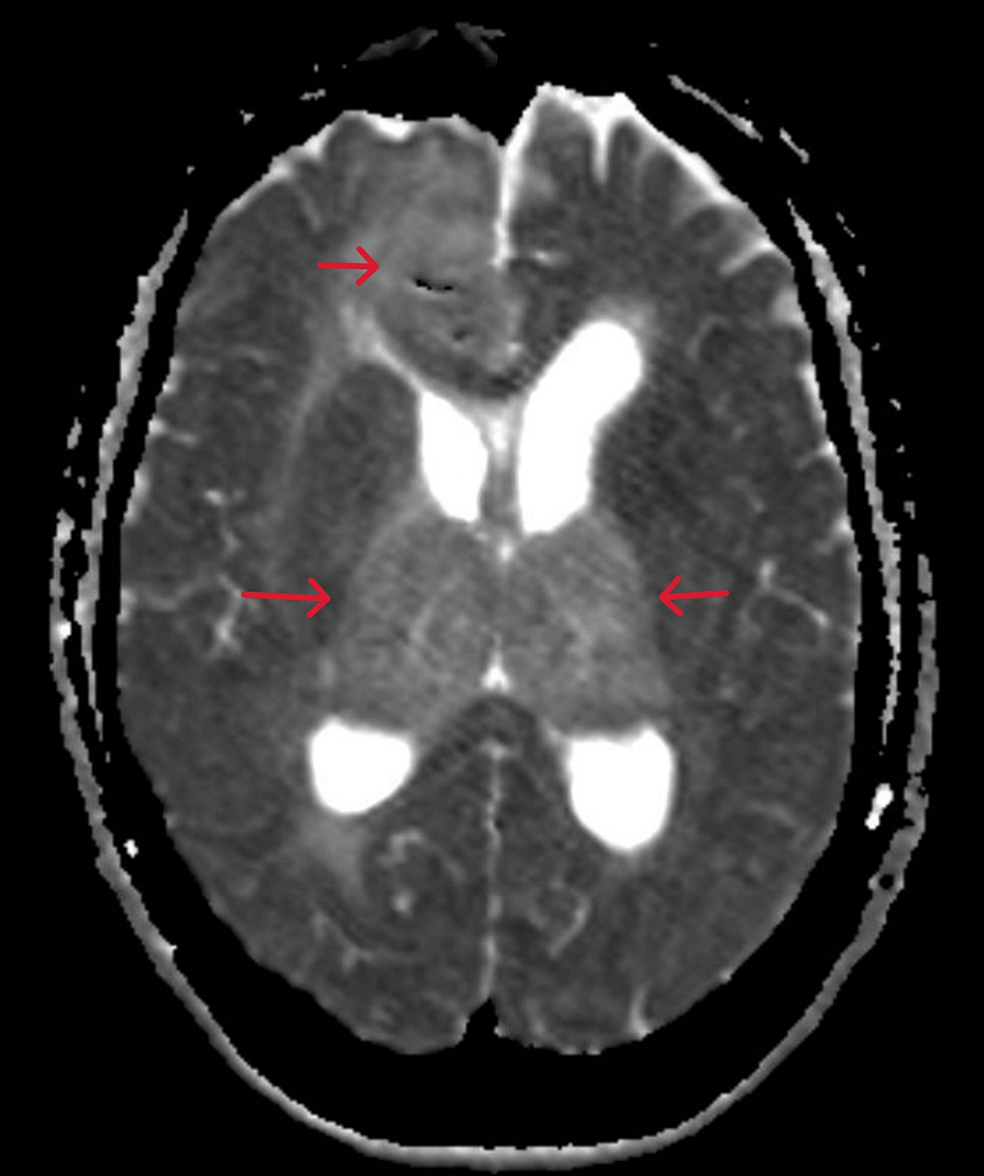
MRI brain T2 - Multiple ill-defined areas of altered signal intensity exhibiting variable T1 signal, low T2 signal noted within/adjacent to abovementioned lesions (diffuse areas of calcifications)
